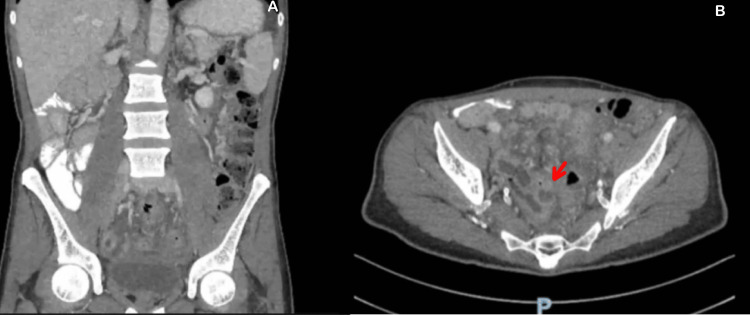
Contrast-enhanced CT scan Demonstrating (A) right-sided small bowel and left-sided colon consistent with intestinal malrotation, and (B) peripherally enhancing tubular structure (arrow) representing an inflamed appendix with associated pelvic abscess.
